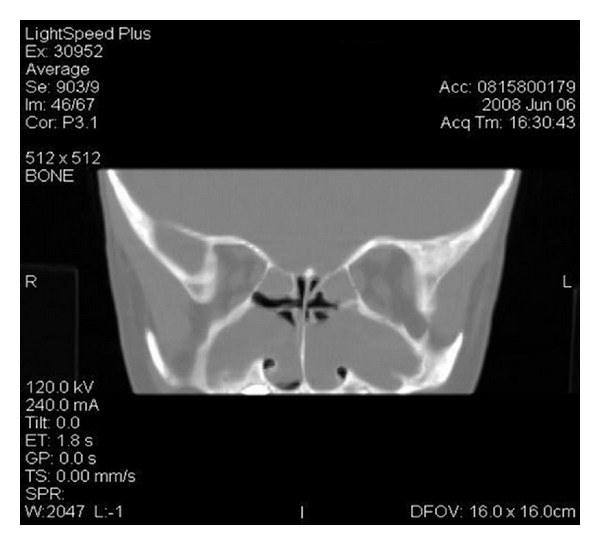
Coronal CT image of bilateral medial displacement of the lateral nasal wall in a pediatric CF patient.
